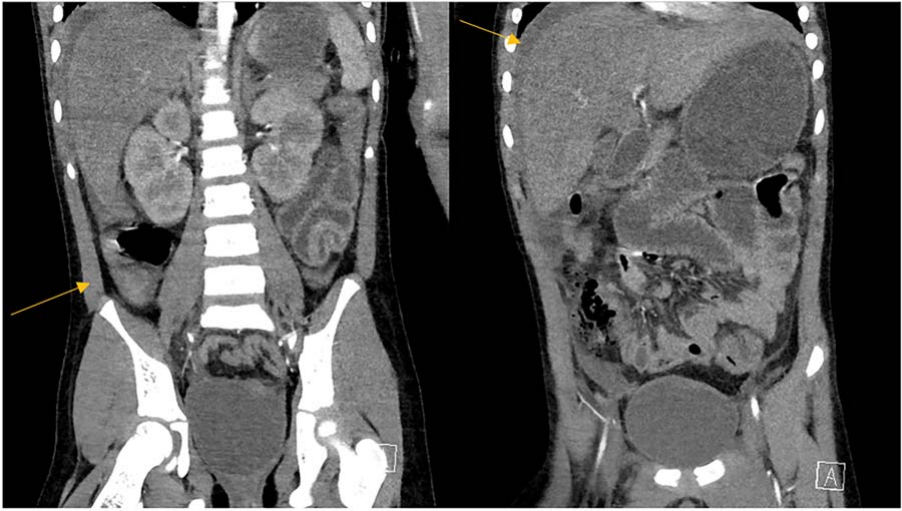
Abdominal CT scan demonstrating intrabdominal free fluid and a collection in the right lower quadrant.
